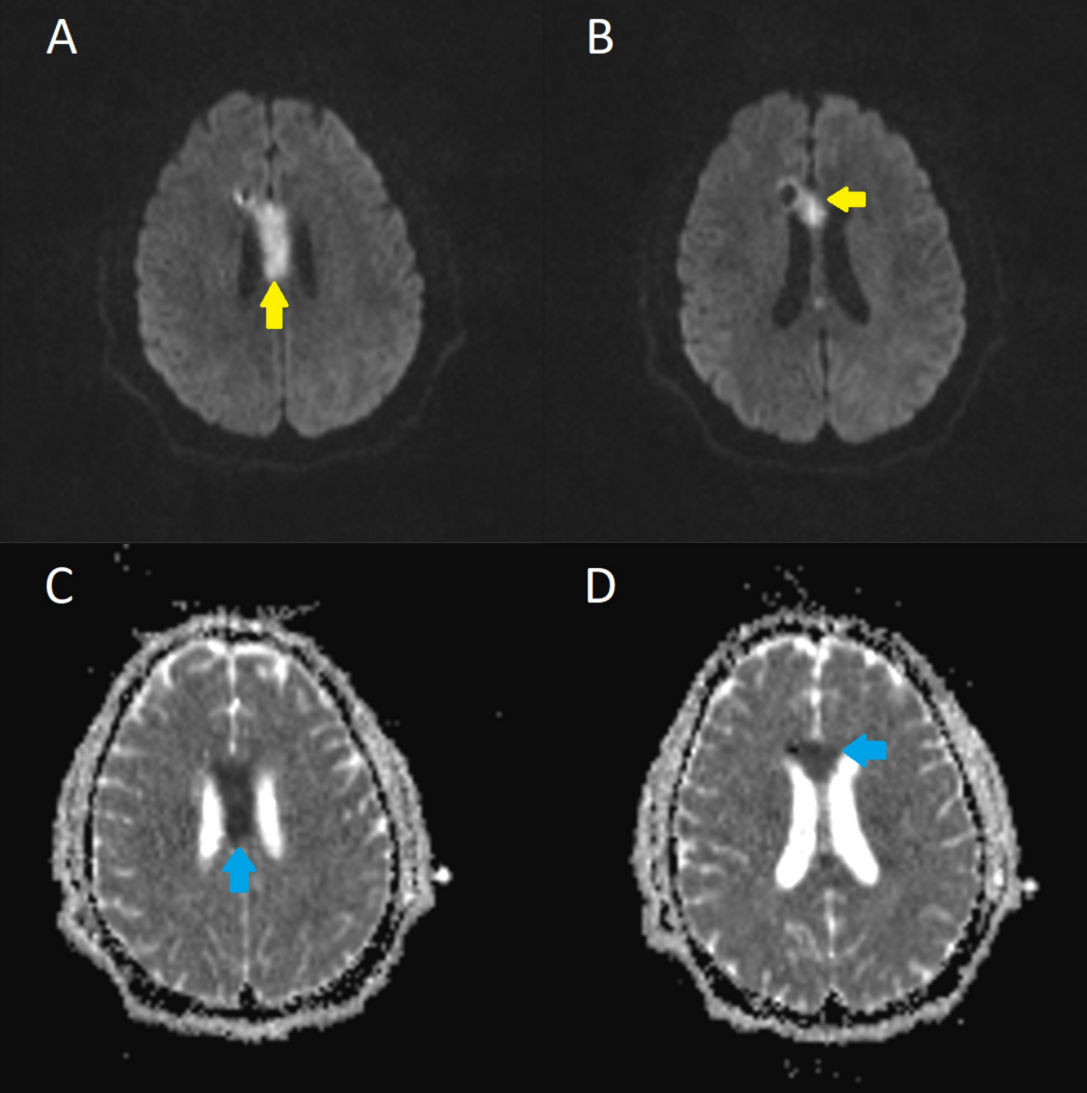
Infarction of the corpus callosum MRI showing areas of hyperintensity in the body (A) and genu (B) of the corpus callosum (yellow arrows) on DWI with restricted diffusion (blue arrows) on respective ADC mapping (C and D), representing a recent ischemic insult DWI: diffusion-weighted imaging; ADC: absolute diffusion coefficient
